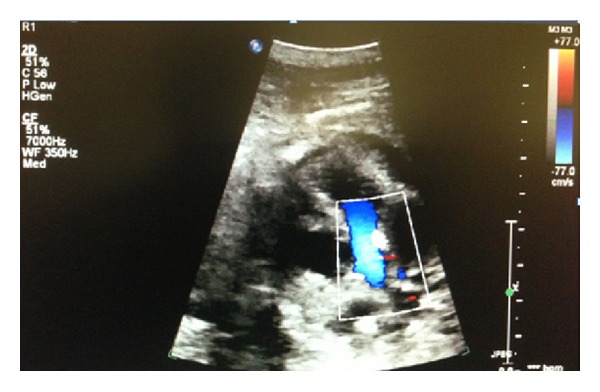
Note the antegrade Doppler flow present in the left ventricular outflow tract.
